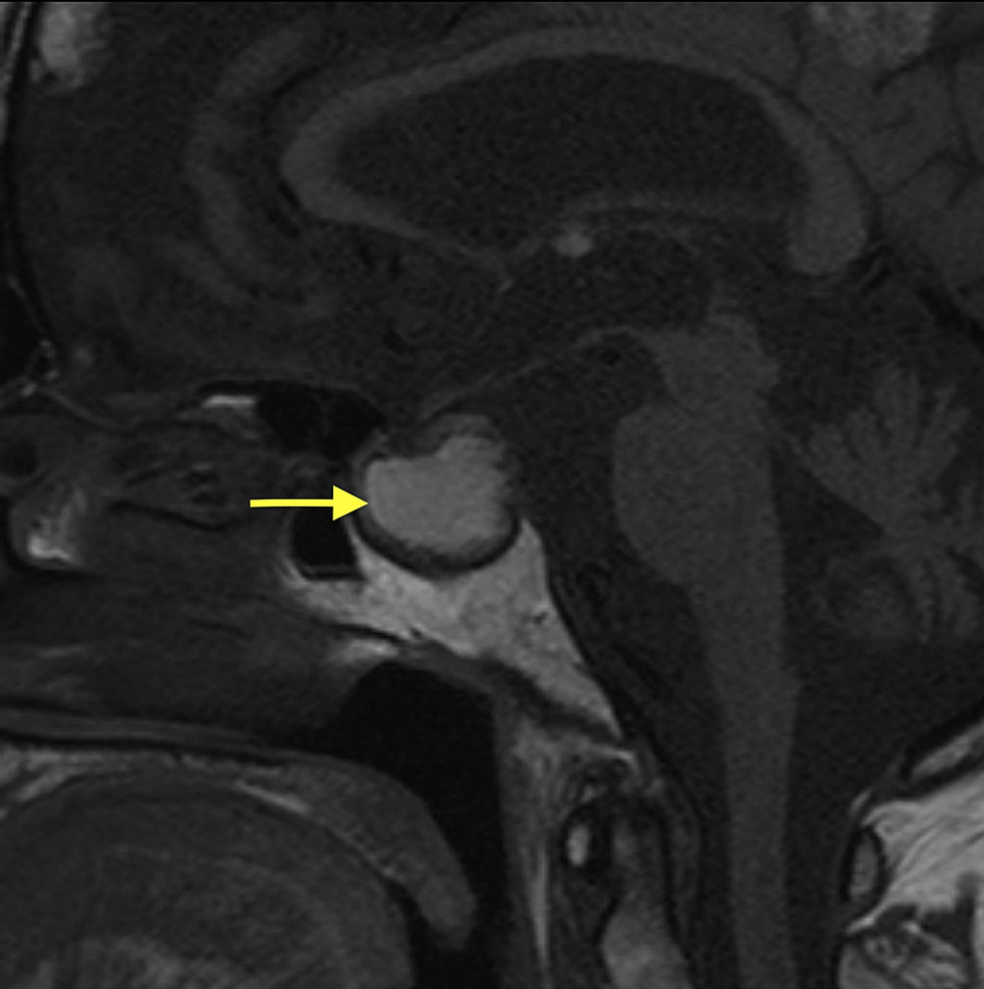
A follow-up MRI revealed reduction in the size of tumor and pituitary bleed (yellow arrow), measuring as 2.1 x 1.9 x 1.8 cm.
